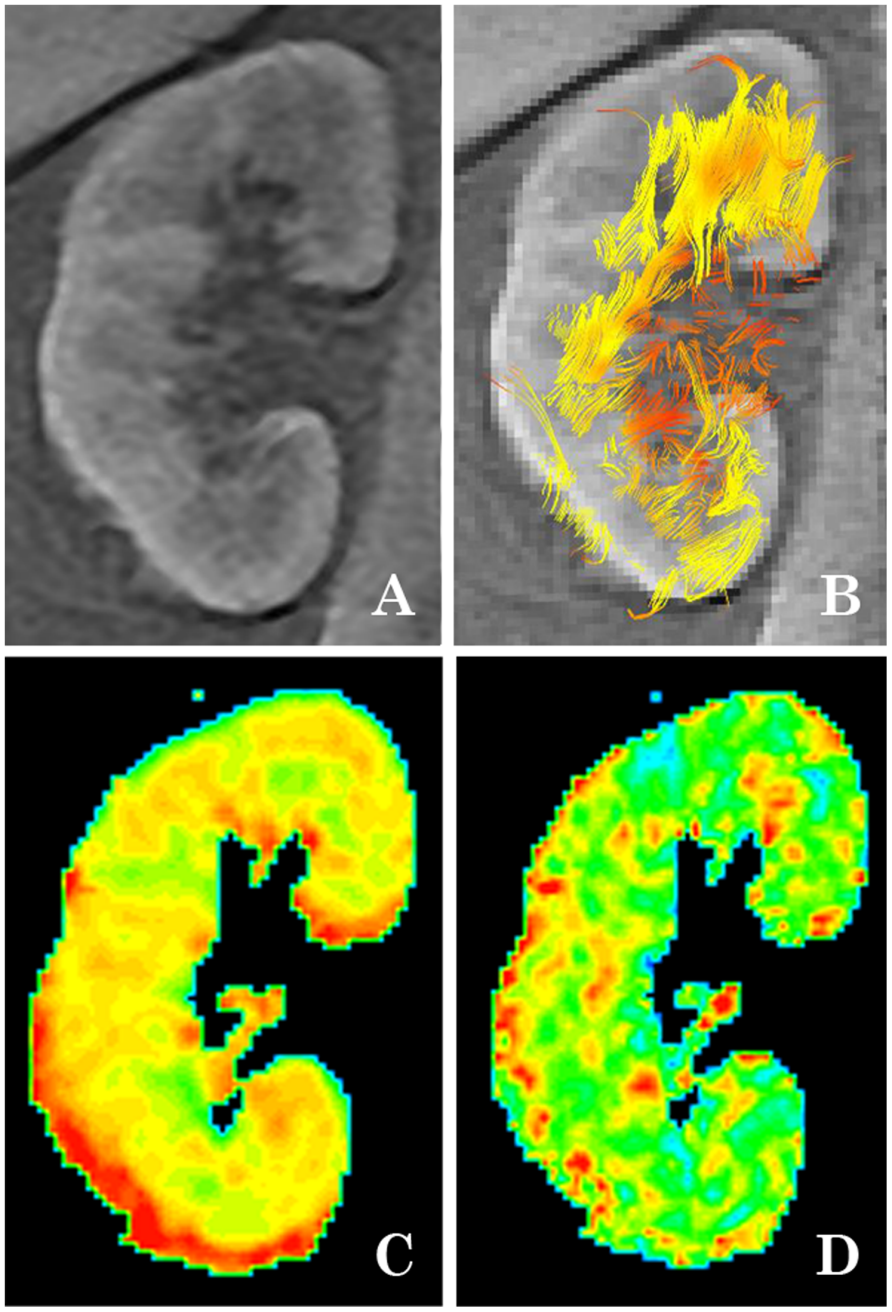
Anatomic and DTI-MRI map of native healthy kidneys. (A) T_1_INPHASE fat-suppression background reference map; (B) DTI tractographic map; (C) DTI MD pseudo-color map; (D) DTI FA pseudo-color map.
